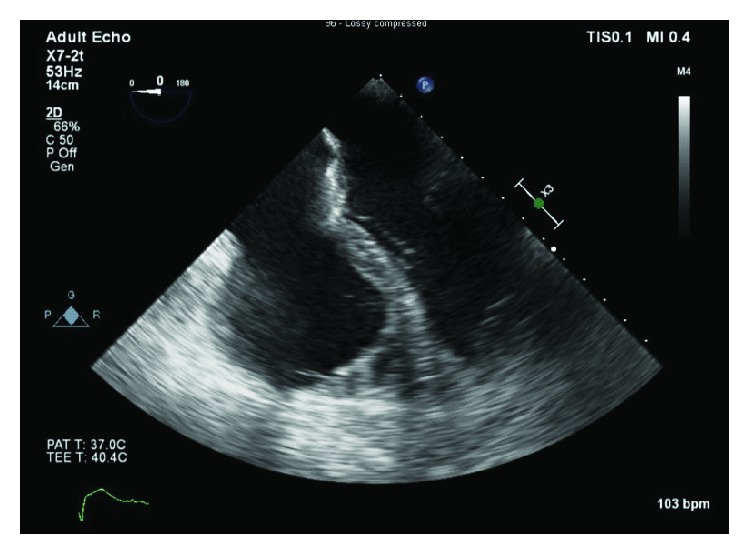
Transesophageal echocardiography post cardiac arrest revealed severe right ventricular dilatation.
